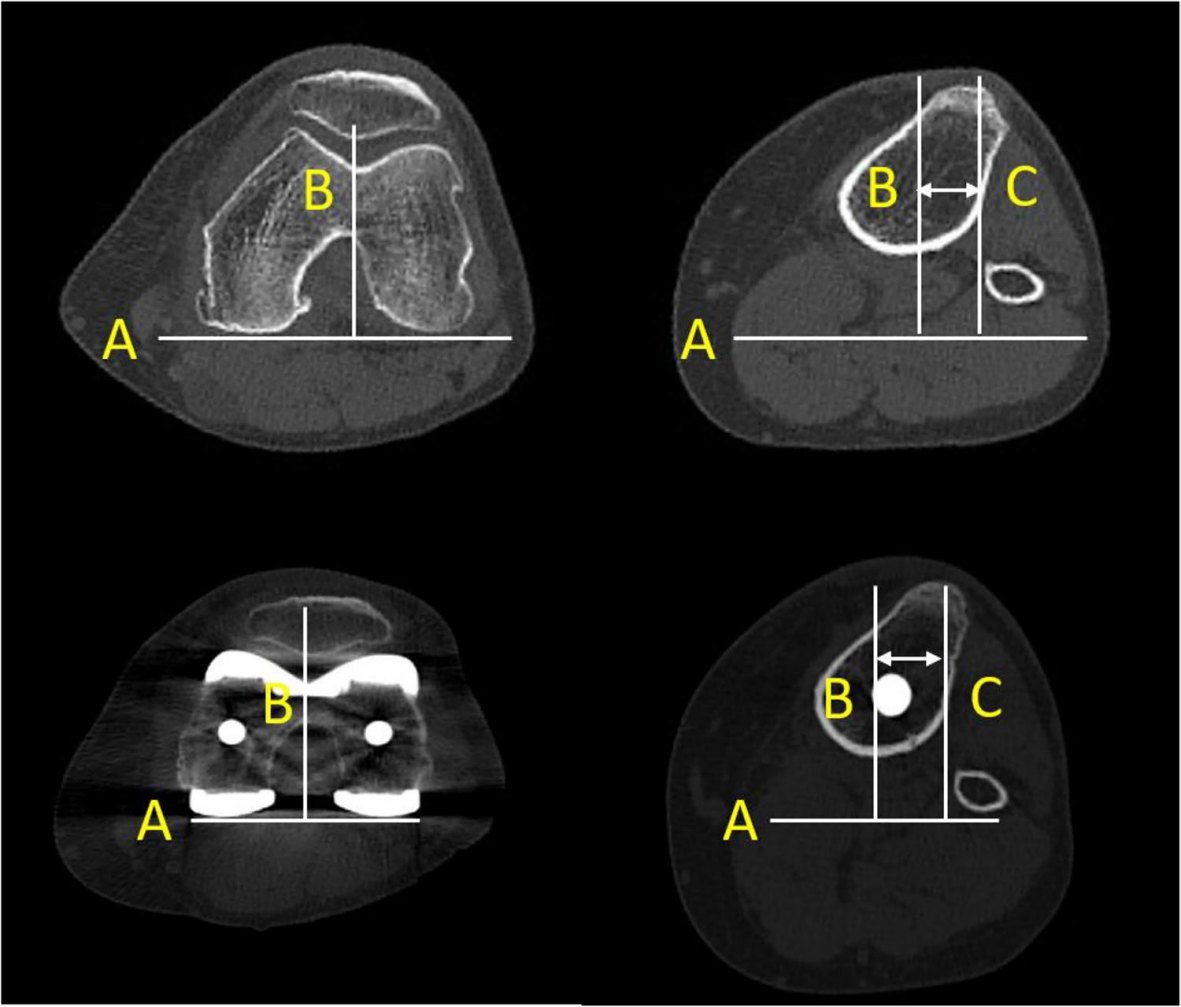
Measurements of tibial tuberosity-trochlear groove (TT-TG) distance pre-operative (above) and post-operative (below). First, the CT scan showing the deepest point in trochlea groove was selected and a tangent to the posterior condyles was drawn (line A). Then, a line perpendicular to line A was drawn through the deepest point of the groove (line B). These two lines were fixed (no change when selecting scrolling through scans), and the scan best showing the tuberosity tibiae was selected. Then, a second perpendicular line to line A was drawn through the most anterior point of the tuberosity tibia (line C). Finally, the distance between these two perpendicular lines was measured
